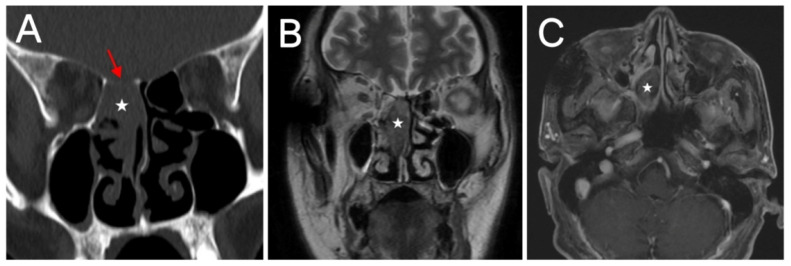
Imaging features of SMARCA4-deficient sinonasal carcinoma (Case 14). (A) Coronal noncontrast CT showing a soft tissue mass in the posterior right nasal cavity (white star), poorly demarcated from the anterior skull base (red arrow). (B) Coronal T2-weighted MRI showing mildly hyperintense signal. (C) Axial post-contrast T1-weighted MRI demonstrating heterogeneous enhancement with indistinct margins relative to adjacent structures.
